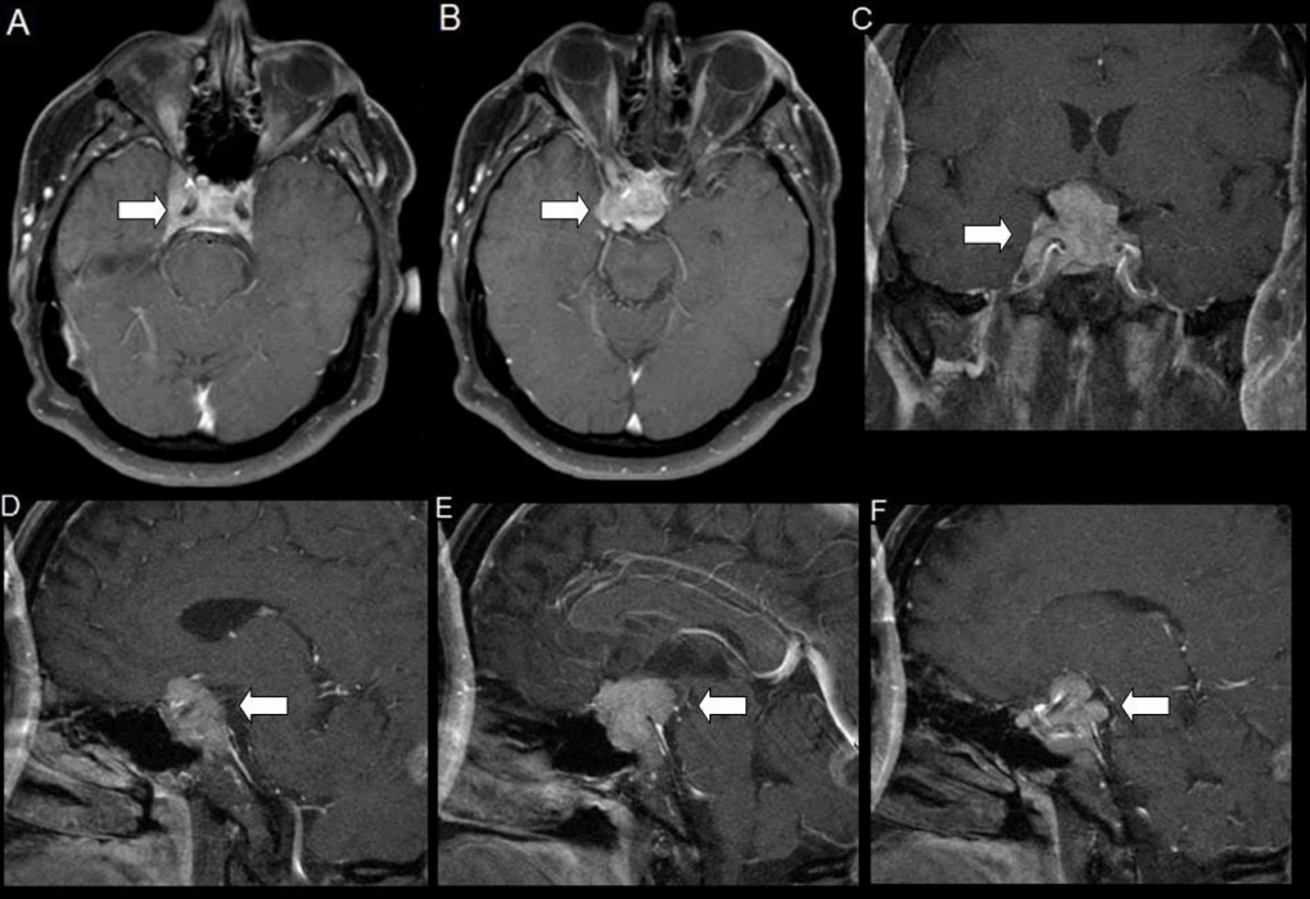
Contrast Enhanced Brain Magnetic Resonance Imaging (MRI) on Presentation Axial (A, B), Coronal (C), and Sagittal (D, E, and F) images showed an enhancing, lobulated mass in the sella arising from the pituitary enveloping the right carotid siphon, right cavernous sinus, optic chiasm, and right optic nerve. The parenchyma was otherwise normal.
